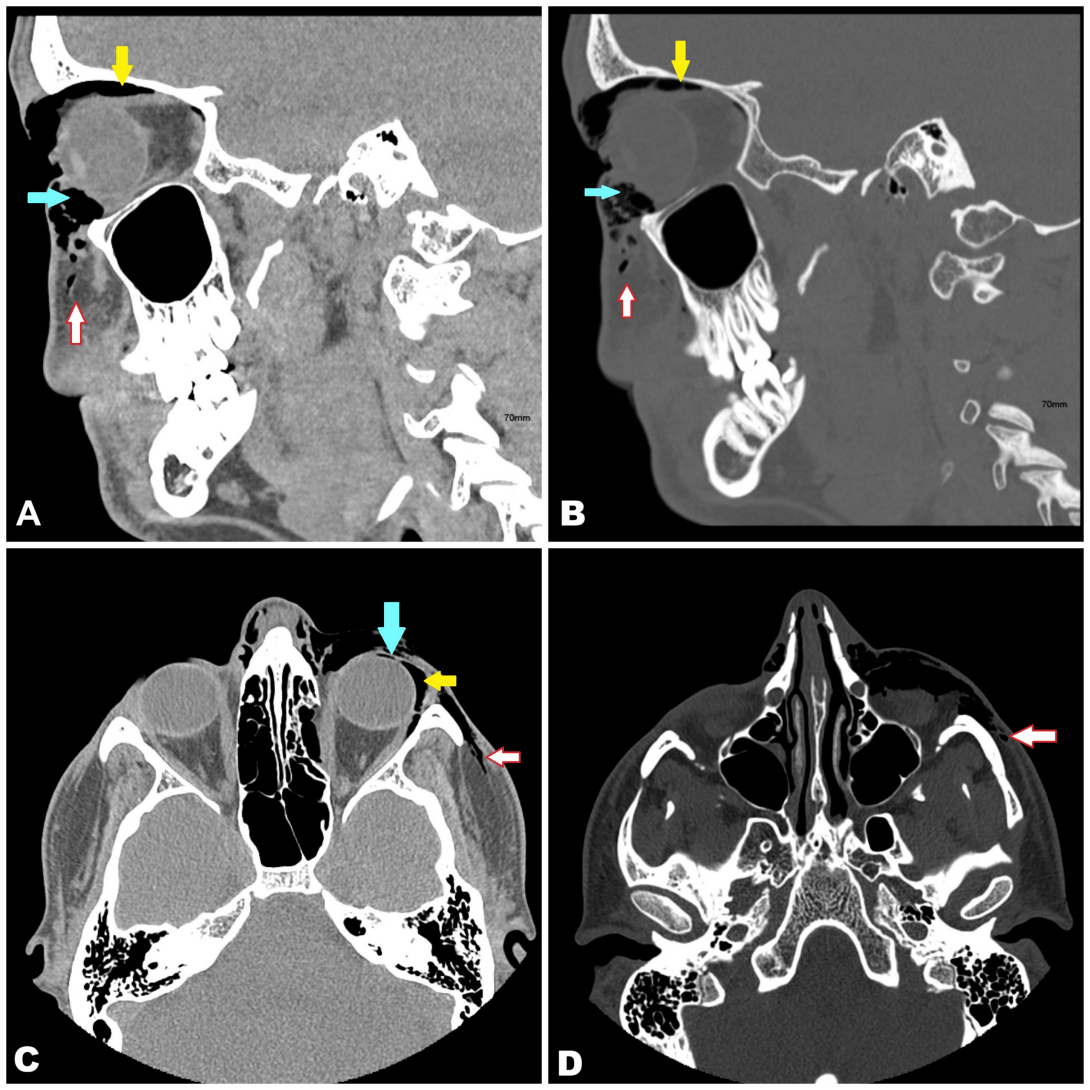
Sagittal (A and B) and axial (C and D) computed tomography (CT) scans of the face demonstrating orbital (yellow arrows), subconjunctival (blue arrows), and subcutaneous emphysema (white arrows) The images highlight the extension of air pockets within the orbital and periorbital tissues.
